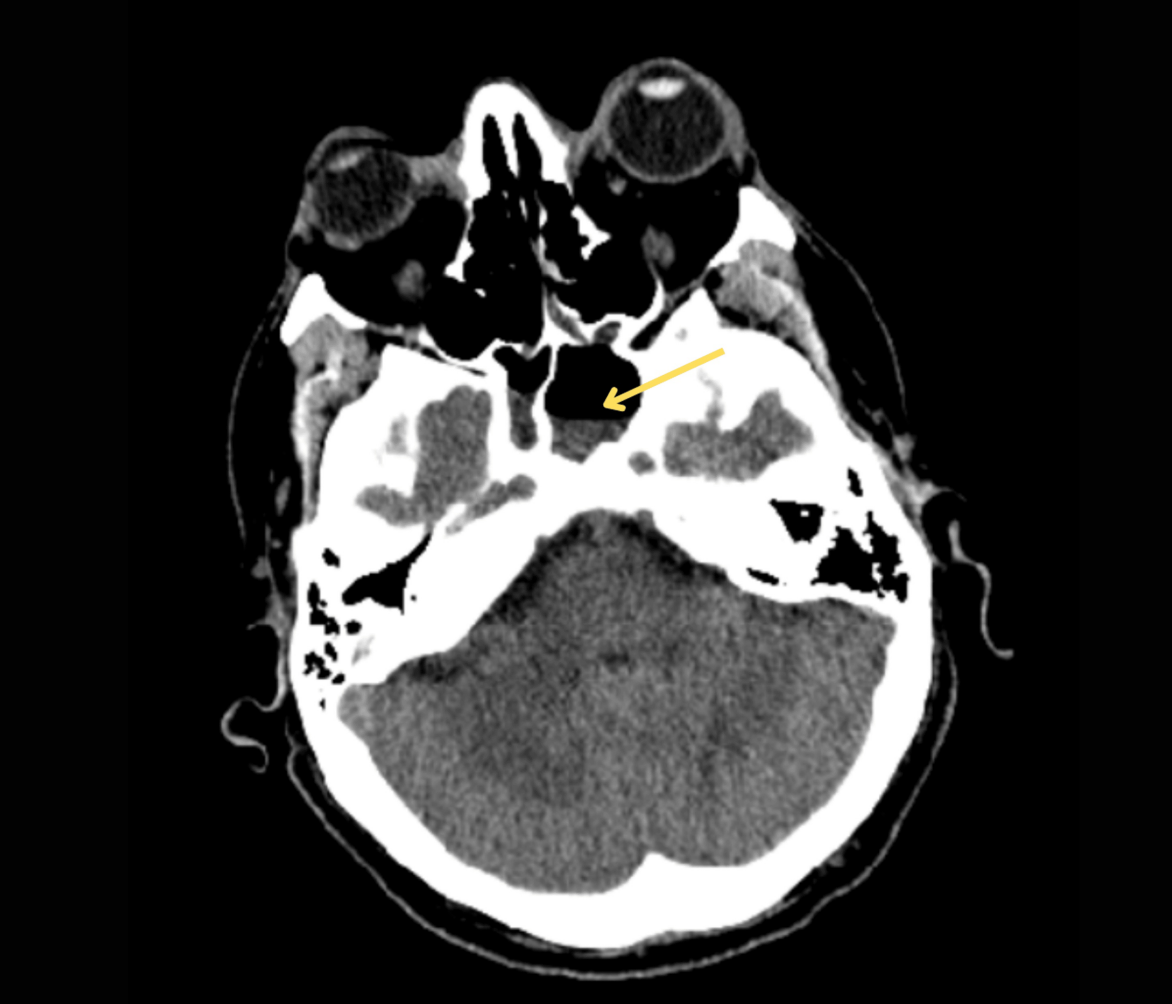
CT brain showing air-fluid levels present within the sphenoid sinuses bilterally
